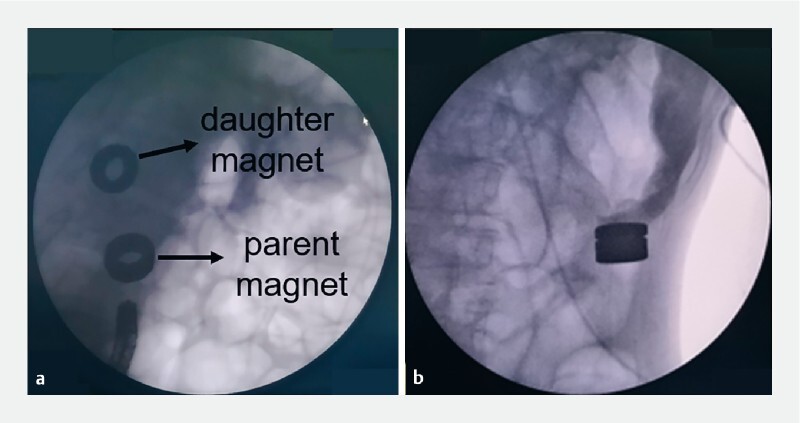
X-ray view of the daughter and parent magnets:
